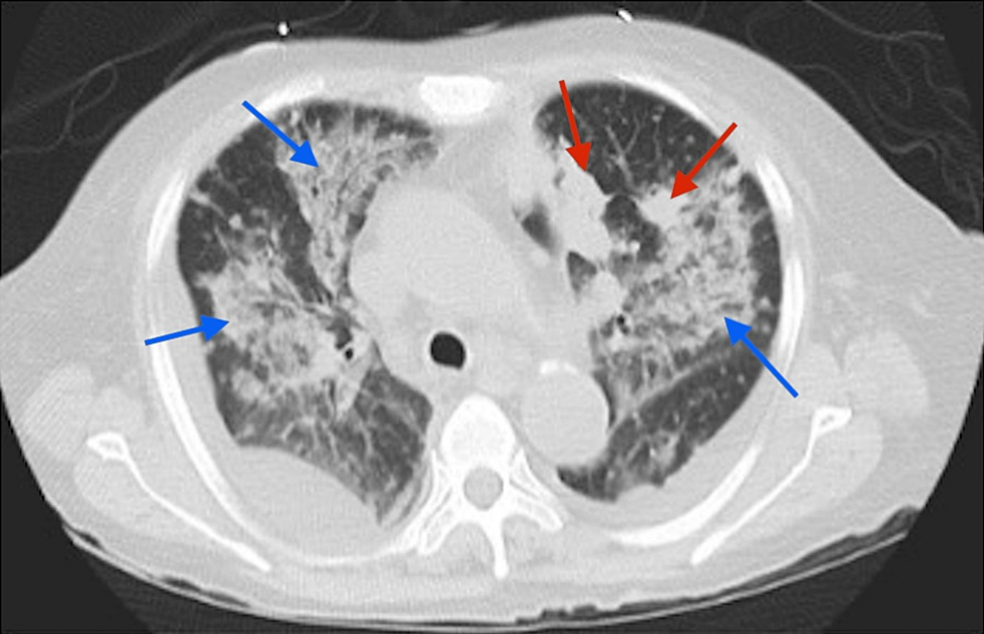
Computed tomography of the chest on day 7 of admission identifying prior nodular opacities (red arrows) and new confluent areas of ground glass opacities with intervening interstitial thickening (blue arrows).
